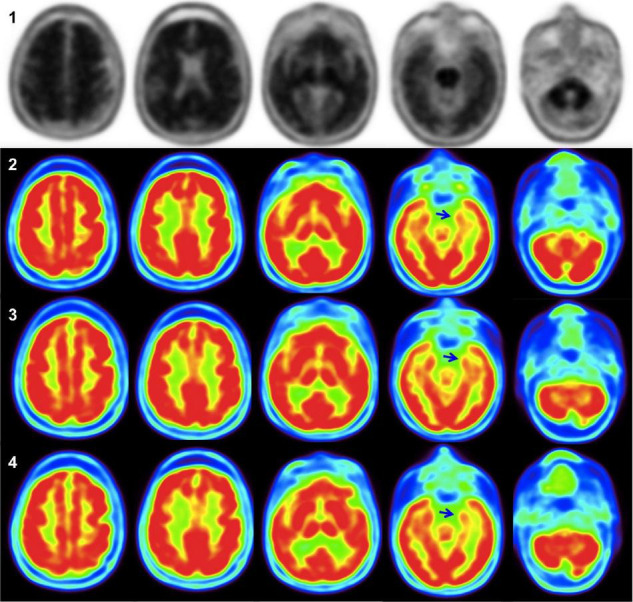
In amyloid PET images (1), the uptake of the grey matter equals that of the white matter in the lateral temporal, parietal, posterior cingulum/precuneus, and especially in the frontal lobe, thus being considered a positive study for amyloid deposition. 18F-FDG-PET images at diagnosis (2) show only mild mesial hypometabolism, predominantly in the left hemisphere (blue arrow). 18F-FDG-PET studies showed no evolution of hypometabolism at 1 year (3), and 2 years after diagnosis (4) (blue arrows).
